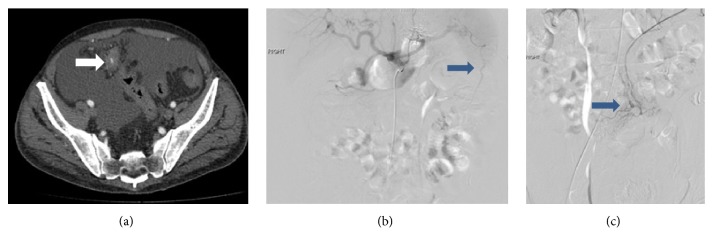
Case 2: Figures 3(a), 3(b), and 3(c). (a) Axial postcontrast CT showing contrast extravasation and surrounding haematoma within the lipomatous lesion (white arrow). Large amount of low density ascities is noted. (b & c): Selective catheterisation of the coeliac axis demonstrated an aberrant artery arising from the splenic artery crossing the midline to the right lower abdomen in the region of the acute haematoma terminating in abnormal cork screw vessels (blue arrows ).
